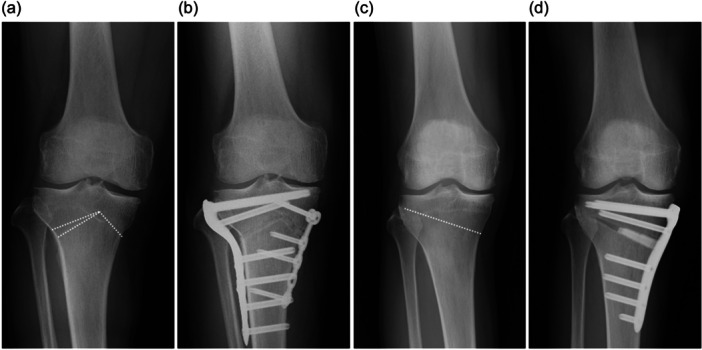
Pre‐ and post‐operative radiographs in HTO. (a) Anteroposterior radiograph before IV‐HTO. (b) Anteroposterior radiograph after IV‐HTO. (c) Anteroposterior radiograph before OW‐HTO. (d) Anteroposterior radiograph after OW‐HTO. Dotted line: osteotomy line. HTO, high tibial osteotomy; IV‐HTO, inverted V‐shaped high tibial osteotomy; OW‐HTO, opening‐wedge high tibial osteotomy.
